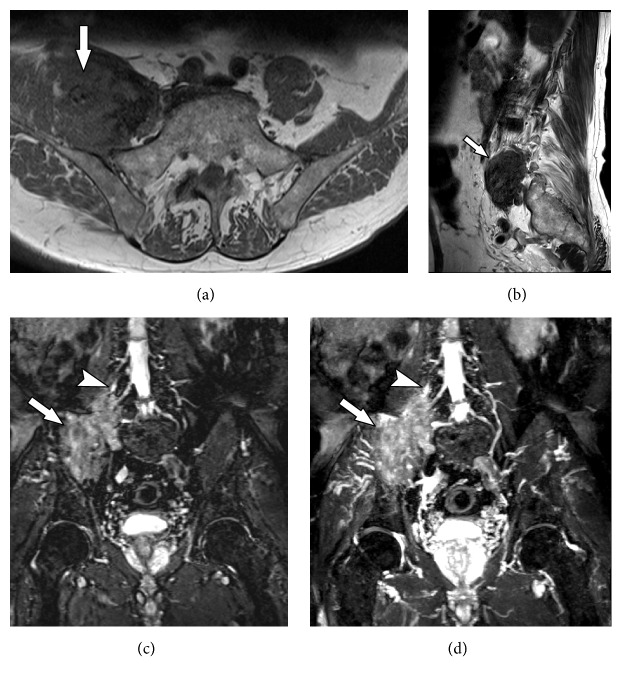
74-years male with low back pain related to a paravertebral tumor. Biopsy revealed an undifferentiated liposarcoma. MRN images in coronal axial T2 (a), sagittal T2 (b), coronal STIR 3D SPACE (c) and coronal STIR 3D SPACE MPR (d) show a heterogeneous solid mass with high T2-weighted signal intensity predominance and contrast enhancement, infiltrating the right psoas muscle in L5 and S1 planes (solid arrow). There is also an extension to the iliac musculature and right lateral aspect of L5 vertebral body, with small insinuation to the neural L5-S1 foramen. The lesion involves the extraforaminal pathway of the L4 root and the proximal portion of the femoral nerve (arrowhead).
